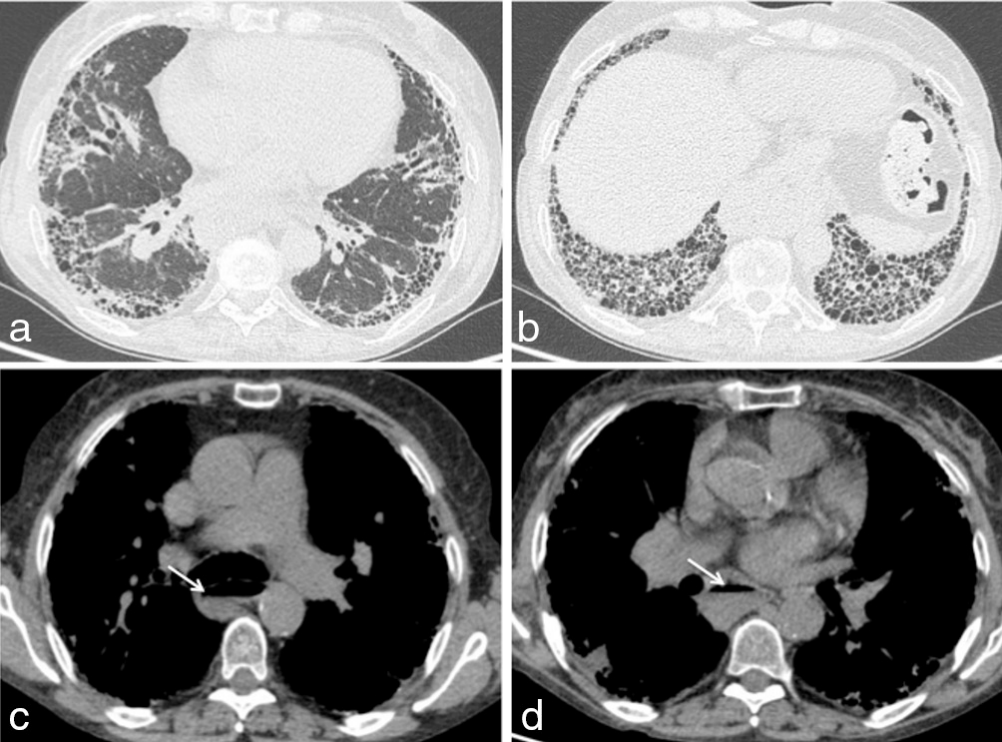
CT scan of the chest. Axial CT images with lung window (a, b) showing signs of bilateral interstitial lung disease with craniocaudal gradient pattern, compatible with pulmonary fibrosis. Axial CT images with soft tissue window (c, d) demonstrating oesophageal dilatation associated with air–fluid level (white arrows).
